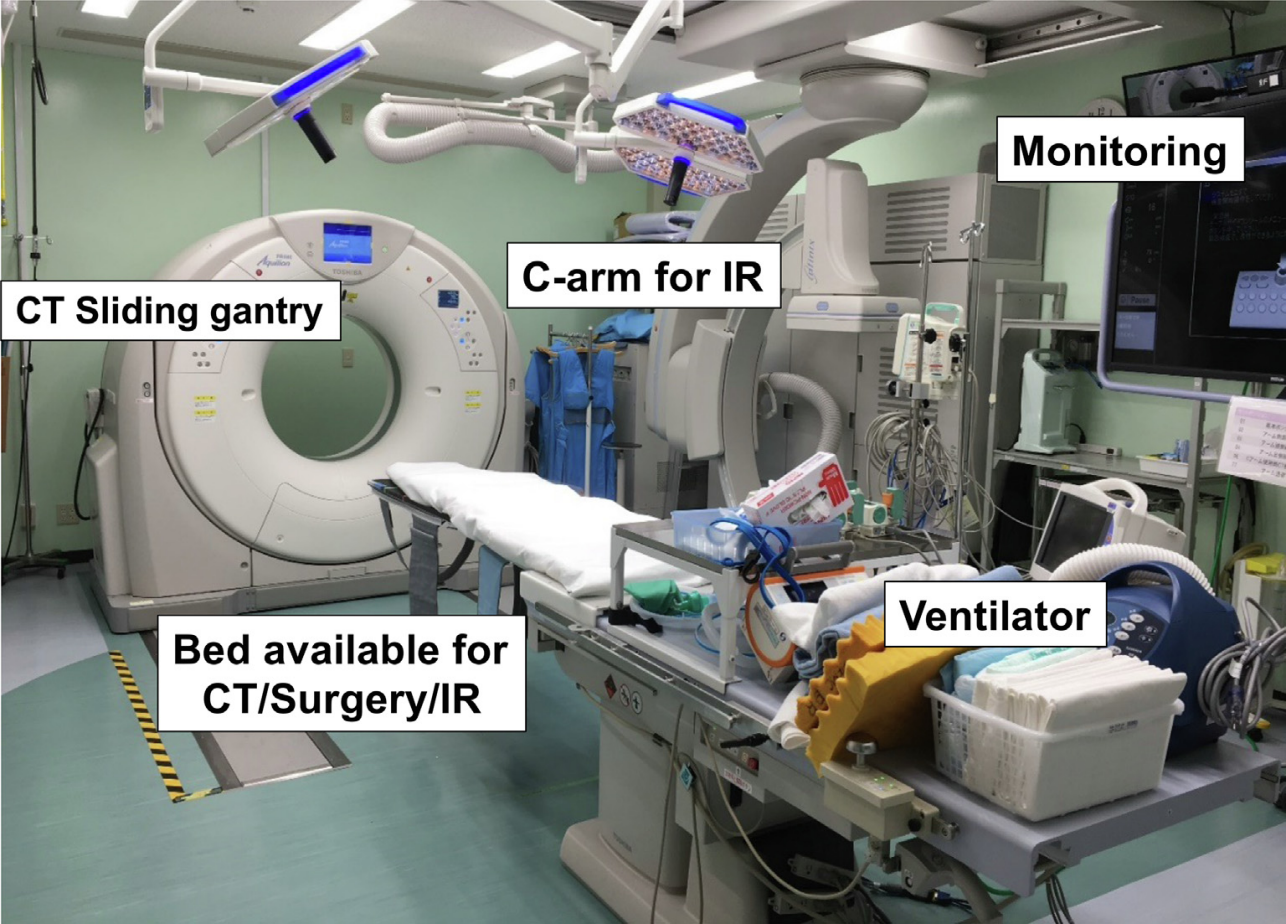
Hybrid ER. All life-saving procedures, including airway management, computed tomography (CT), emergency surgery, and interventional radiology (IR) can be performed promptly and safely on the same bed without transferring patients to other departments.
